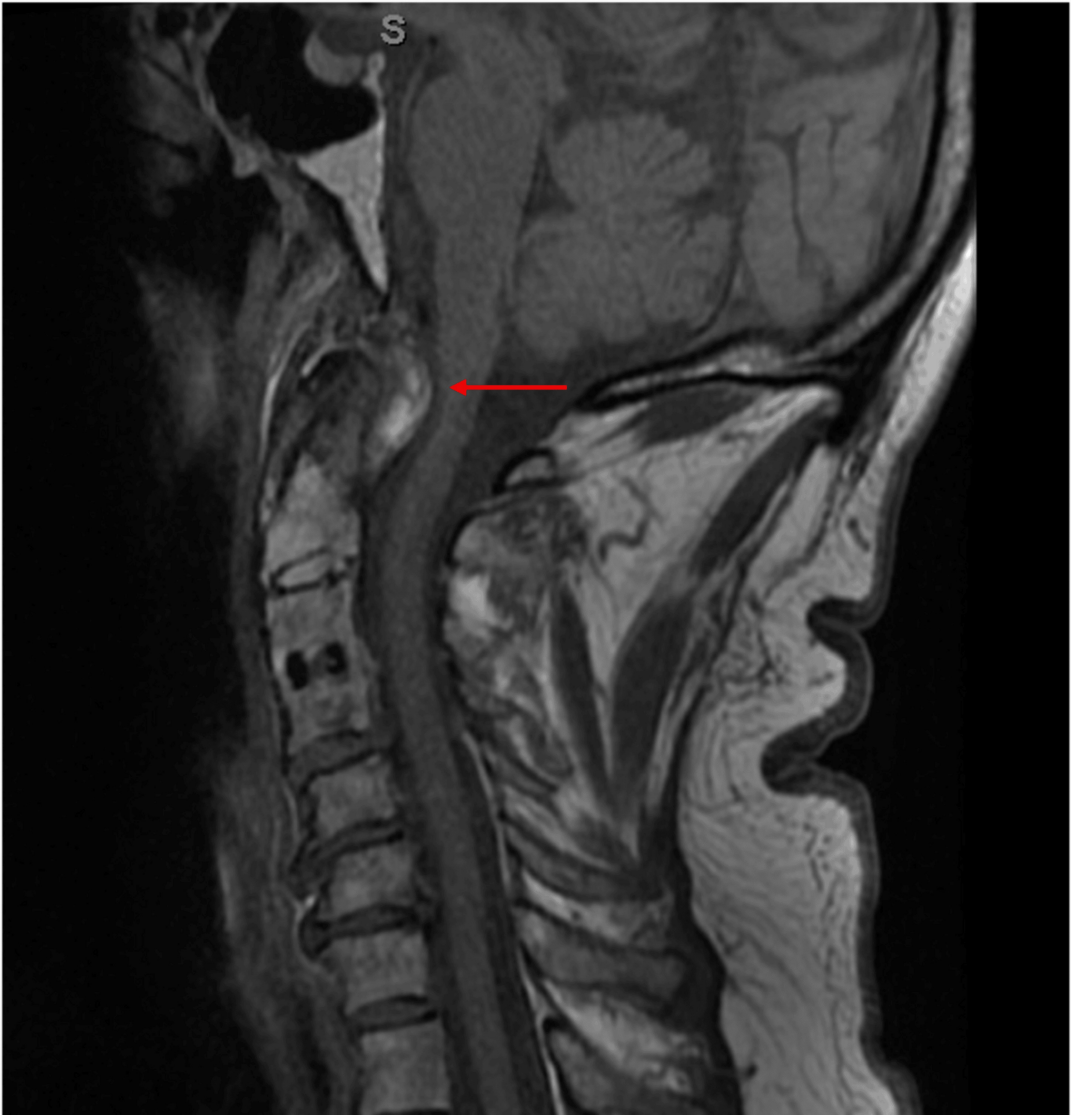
T1-weighted sagittal MRI of the cervical spine. The red arrow indicates pannus formation at the level of the dens.
